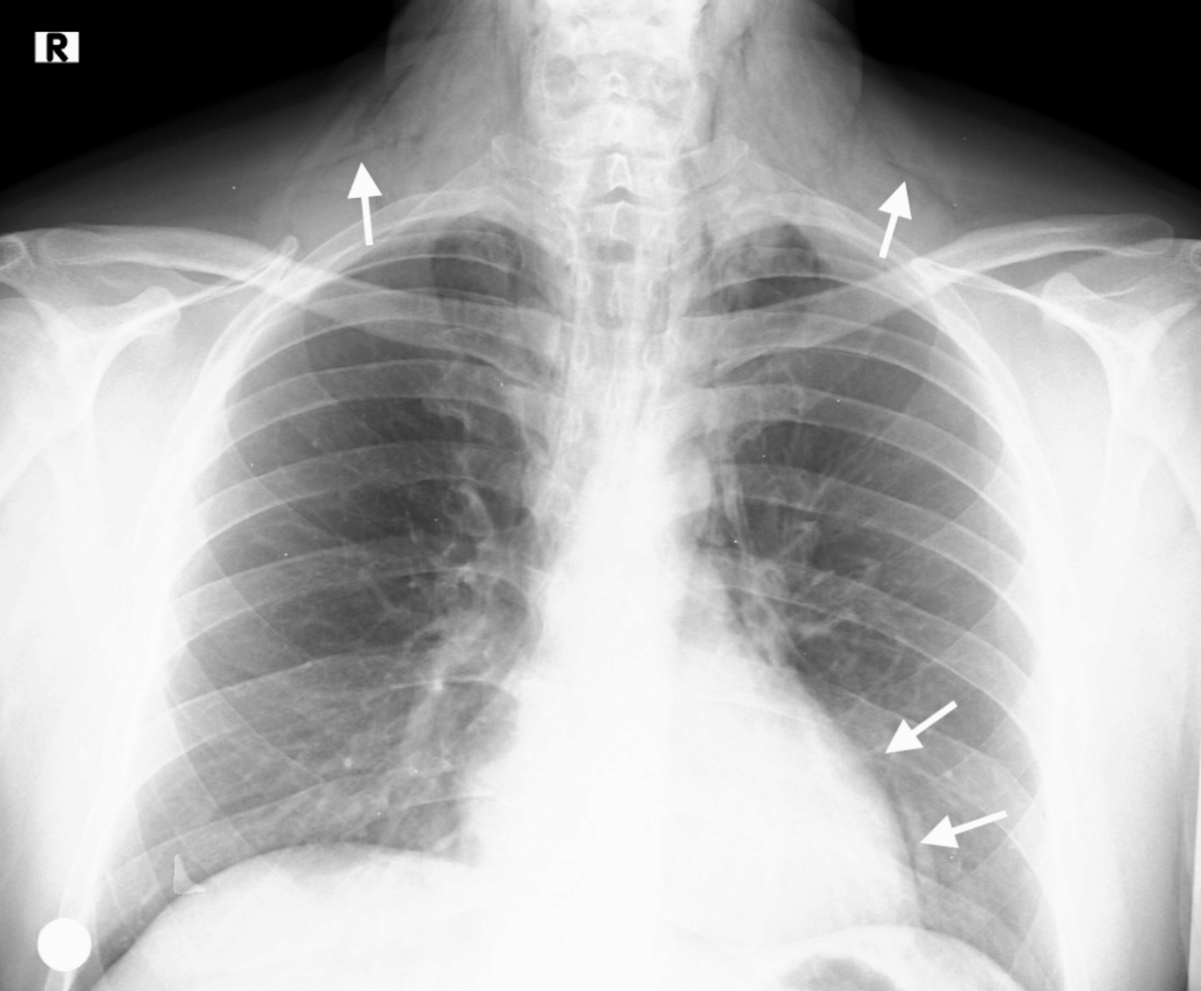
Postoperative thirty-sixth-hour chest X-ray Subcutaneous emphysema can be seen in the neck area and pneumomediastinum in the paracardiac region (white arrows).
